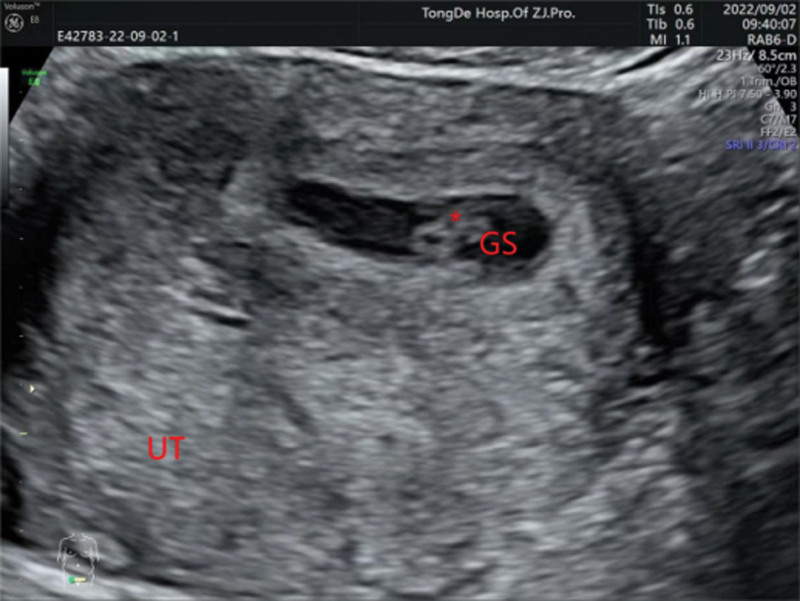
Gestational ultrasound after 3 days of combined treatment (6 weeks, 6 days).
